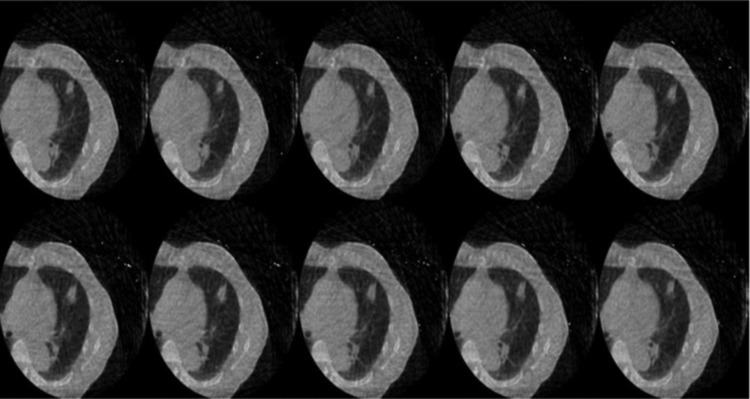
Reconstructed 4D-CBCT (10 phases) for patient data.
